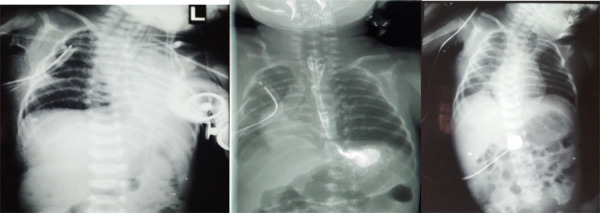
(A) Post-operative X-ray showing atelectasis of left lung and chest tube in right lung. (B) Fluoroscopic evaluation demonstrating patent oesophagus without mediastinal spillage of dye and bilateral normal expansion of lungs field. (C) X-ray demonstrating proximal oesophageal dilatation revealing post-surgical oesophageal stricture.
